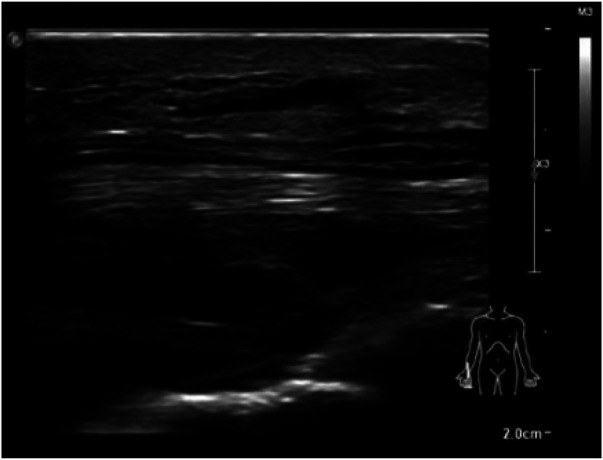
Preoperative ultrasonography examination.
